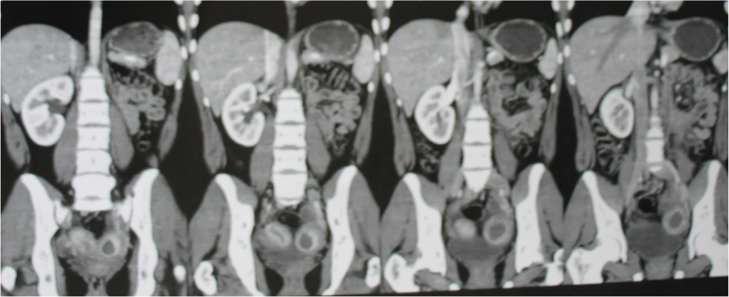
CT demonstrating absent left kidney, uterine duplication, suggestive of cervical duplication, and distension of the left cavity by hypodense material.
